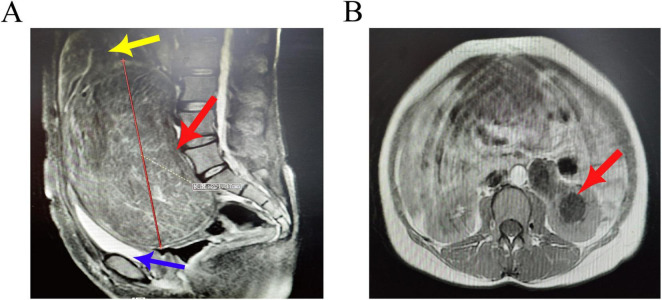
(A) Sagittal view of the pelvic MRI shows a large mass posterior to the cervix with well-defined margins, measuring approximately 20 cm. Multiple rounded short T2 signal lesions are observed in the myometrium, exhibiting mild enhancement (red arrow). The uterine body is elevated due to pressure, and there is elongation of the cervical canal (yellow arrow). The bladder is compressed and displaced anteriorly and downward (blue arrow). (B) Axial view demonstrates dilation of the left renal pelvis and ureter (red arrow).
